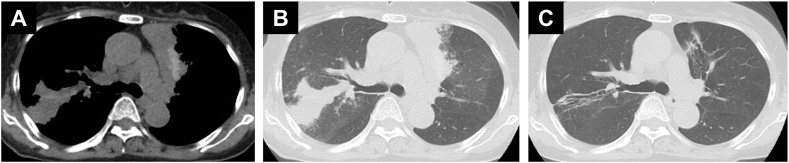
(A, B) Chest computed tomography at the first examination revealing an infiltration with high-attenuation mucus plugs in the bilateral upper lobes. (C) After nine weeks of steroid treatment, chest computed tomography revealing no evidence of infiltration and mucus plugs.
